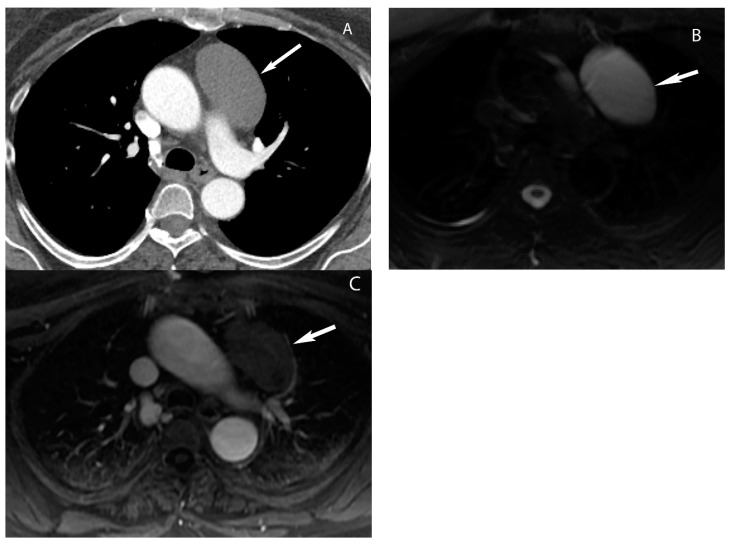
Thymic cyst. (A) CT shows a well-defined homogeneous structure with density slightly higher than simple fluid (40 Hounsfield units) in the prevascular mediastinum (arrow). MRI (B,C) shows T2 hyperintensity (arrow in (B)) and no enhancement with gadolinium (arrow in (C)) consistent with fluid.
